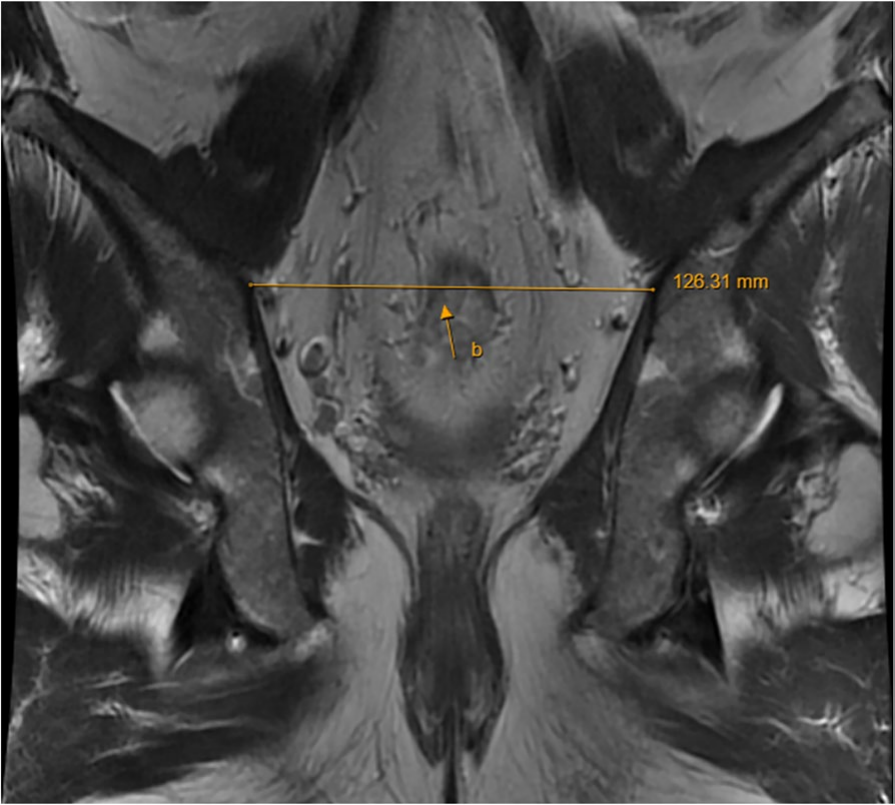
Coronal MRI of the pelvis: b pelvic entrance transverse diameter
